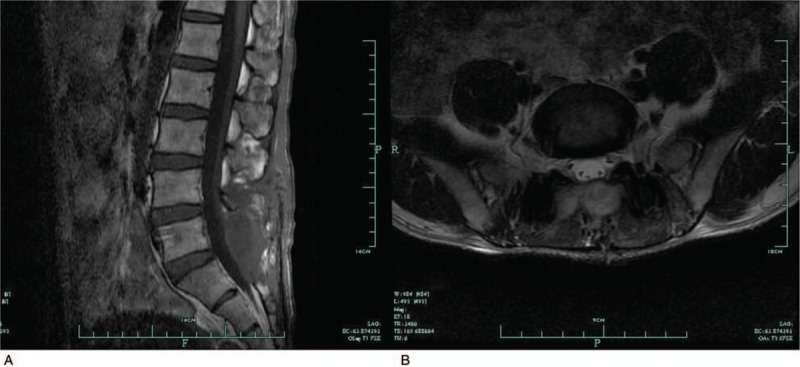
Post-operative MRI images. Sagittal T1-weighted (A) and axial T2-weighted (B) images showed the uncompressed cauda equina. MRI = magnetic resonance imaging.
